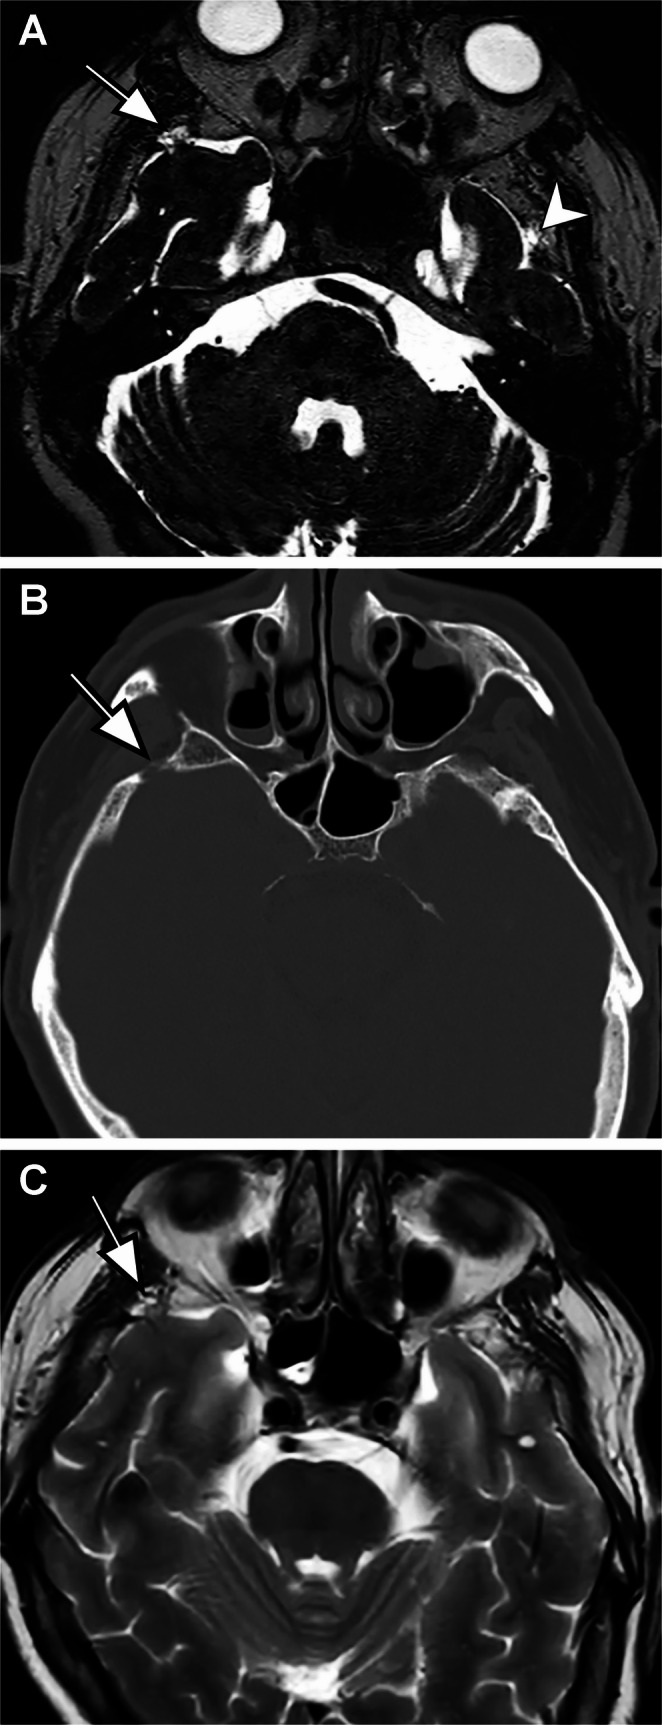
Forty-nine-year-old male with tinnitus. A Axial 3D-DRIVE image demonstrates that right anterior temporal lobe herniation (arrow) and left anterior temporal pit without herniation (arrowhead). B Axial head CT image (bone window) shows bone defect (arrow) at the level of herniation. C Note that herniation (arrow) is barely discernible on the axial T2-weighted MR image
